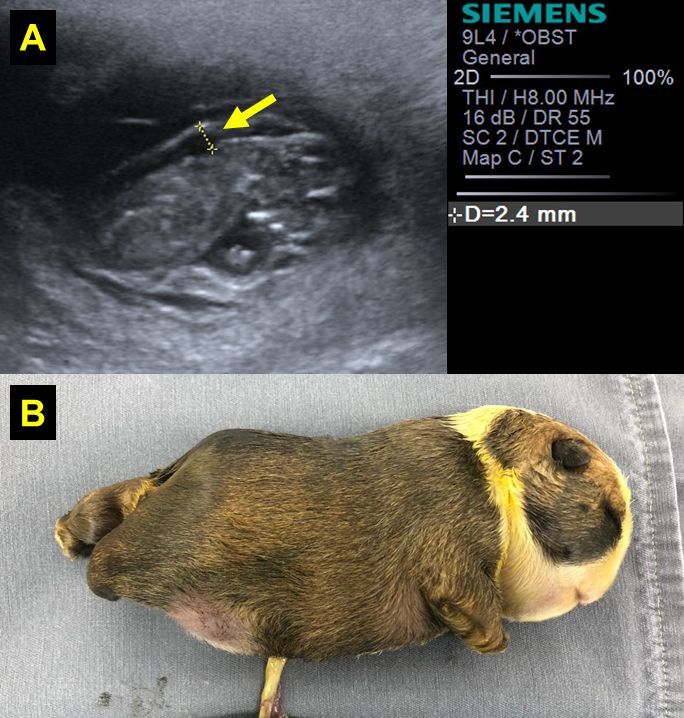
(A) An ultrasonography image of increased NT (nuchal translucency - arrow) measurement (NT = 2.4mm) in a canine fetus, at 34 days of gestation; (B) Fetus of image A, exhibiting anasarca at birth.
